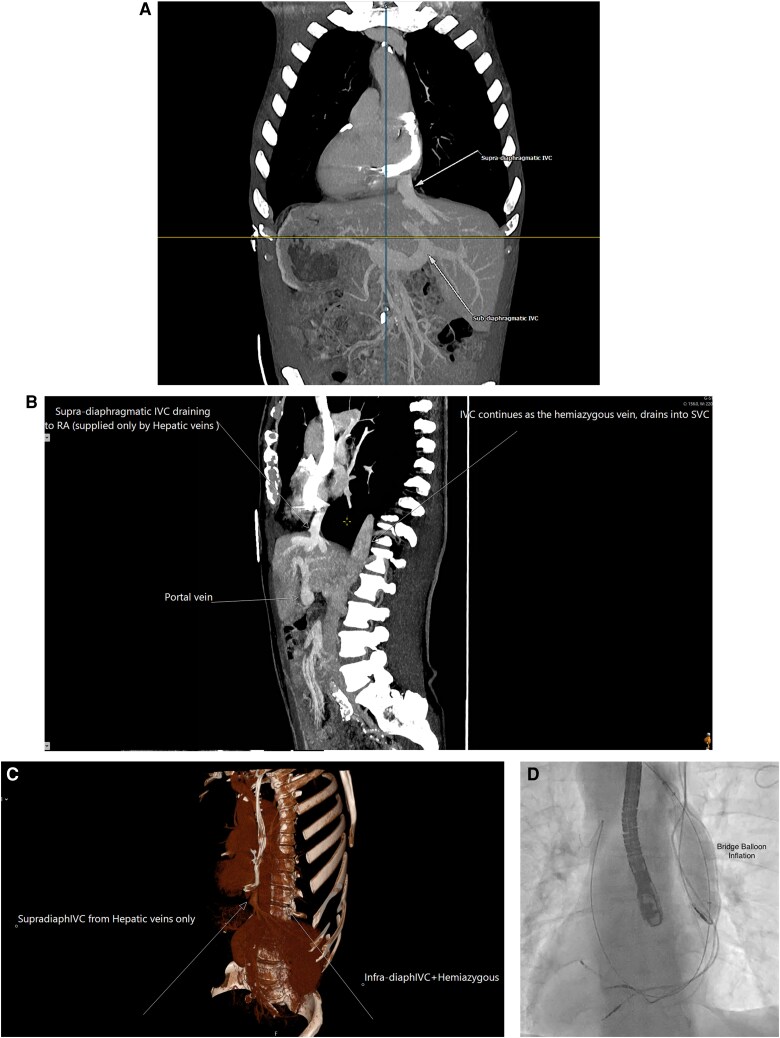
(A-C)* Annotated computed tomography imaging demonstrating dextrocardia with interruption of the IVC. *(D)* Bridge Balloon delivered using superior venous approach.
